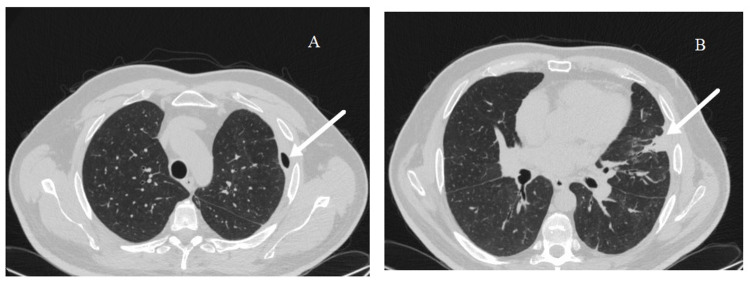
Control CT of the chest after two months. (A) CT showing marked resolution of the pneumothorax (arrow). (B) CT showing the healed bronchopleural fistula (arrow).
